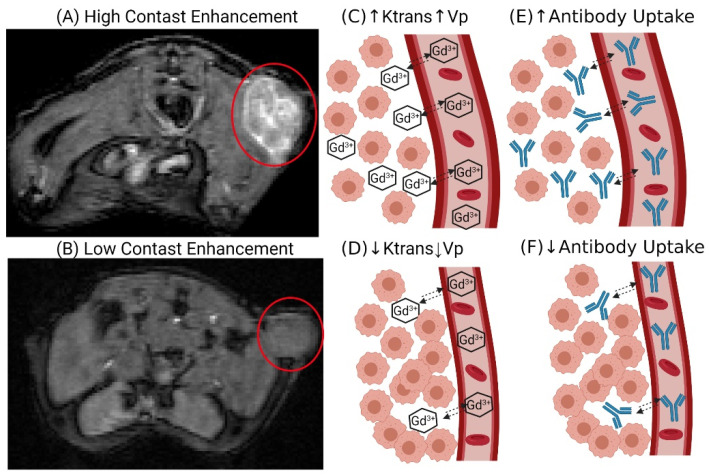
DCE-MRI: The DCE-MR images on the left side provide examples of a tumor with high contrast enhancement (A) and low contrast enhancement (B) following gadobutrol injection. High contrast enhancement is the result of greater gadobutrol uptake into the tumor (C), which results in larger values for Ktrans and Vp relative to the low contrast-enhancing tumor (D). The DCE-MRI parameters are representative of tumor physiology (vascular permeability, plasma volume, and blood flow) that is known to impact mAb disposition; therefore, the high contrast-enhancing tumor is expected to have greater mAb uptake (E) relative to the low contrast-enhancing tumor (F). Created with BioRender.com (accessed on 23 Novermber 2021).
